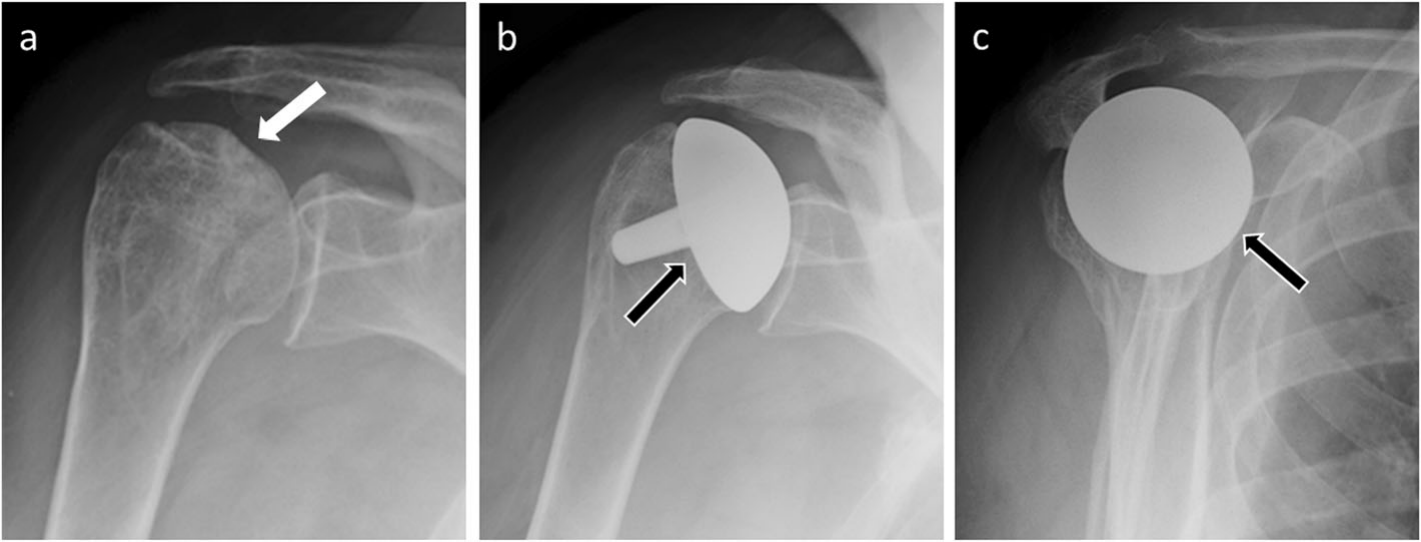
a Anteroposterior radiograph in a 63-year-old woman shows avascular necrosis of the humeral head (white arrow). Anteroposterior (b) and scapular Y (c) radiographs of normal findings in the same patient as in a with humeral head resurfacing arthroplasty. The humeral prosthetic head (black arrows) is centered within the native glenoid
